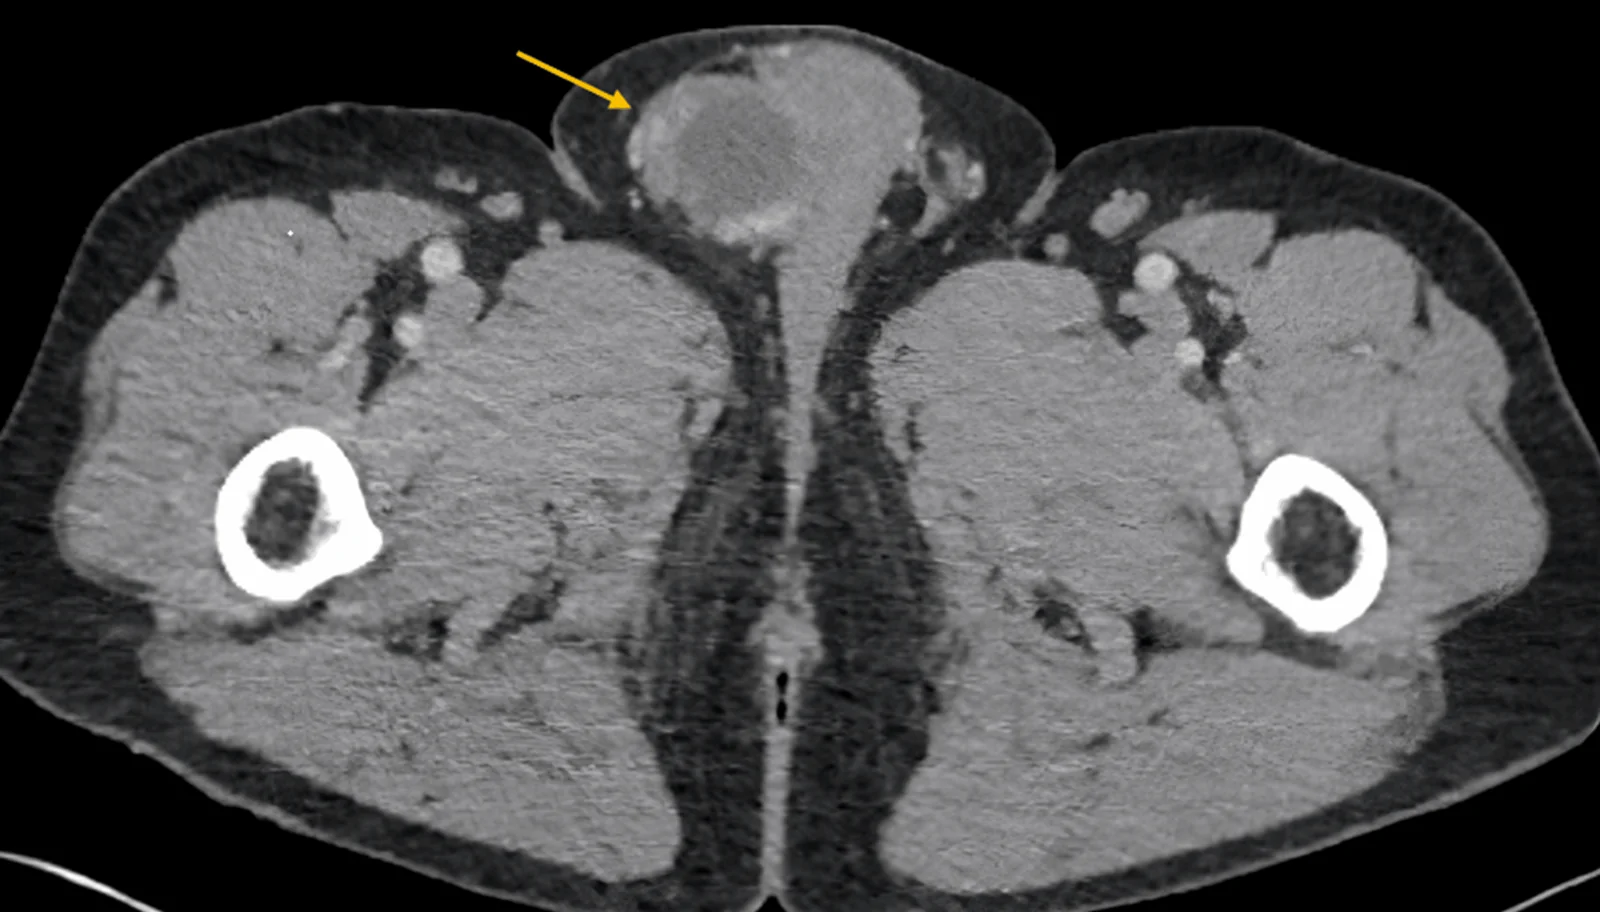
Contrast-enhanced axial CT image showing serpiginous enhancing soft tissue in the right hemiscrotum (yellow arrow), representing malignant mesothelioma of the tunica vaginalis.
